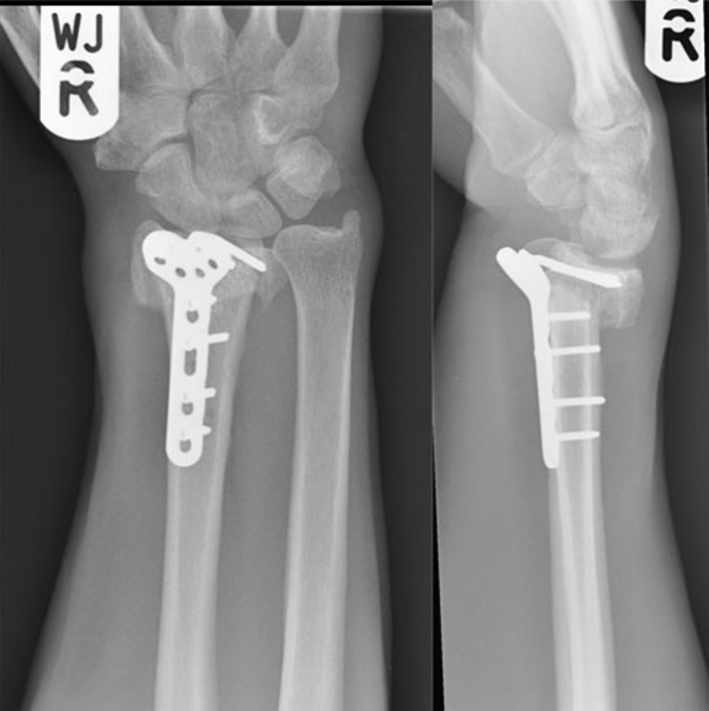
Radiographs at 26 weeks after the first revision procedure. Broken screws
